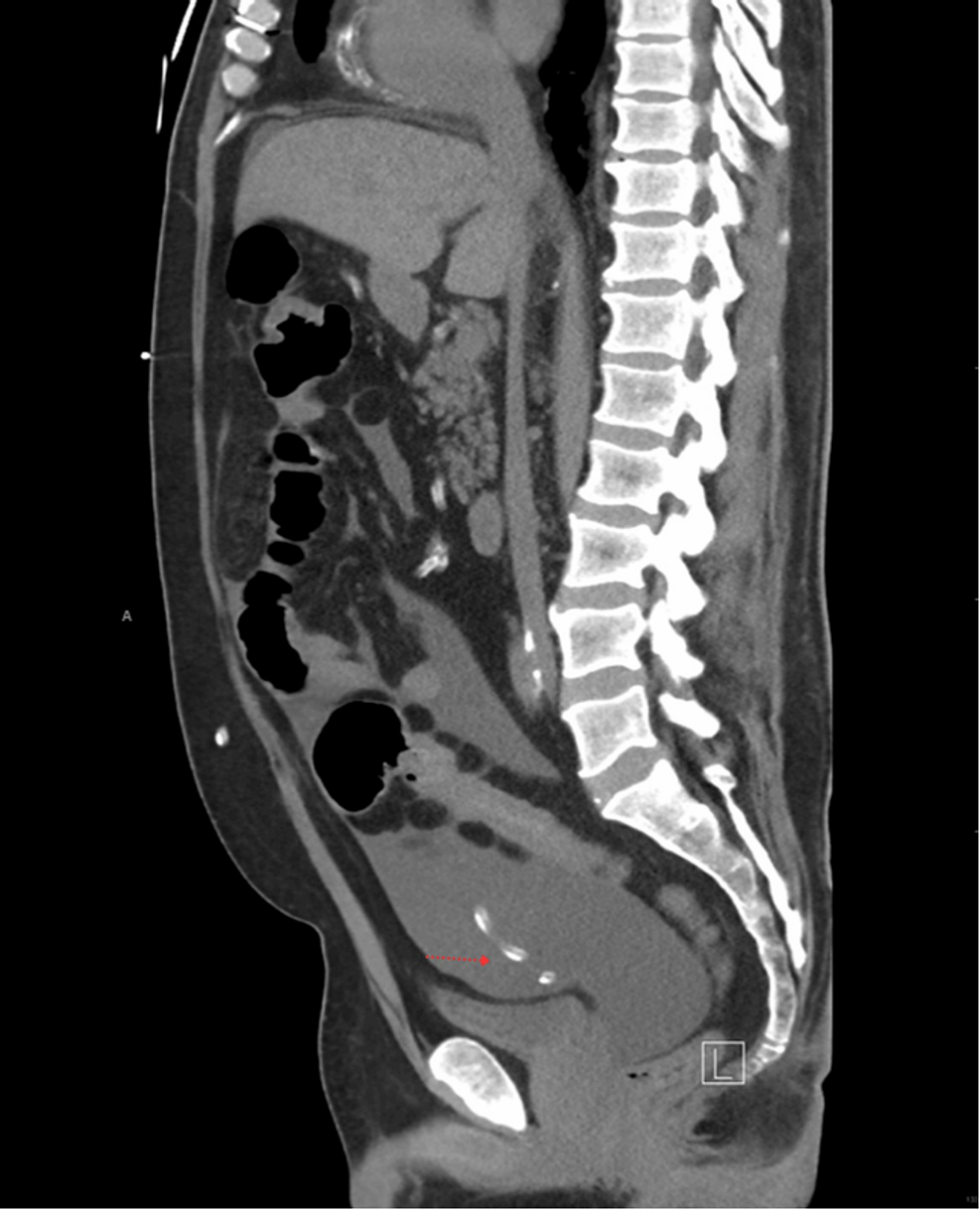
Sagittal view of CT abdomen and pelvis showing peritoneal fluid and peritoneal catheter.
